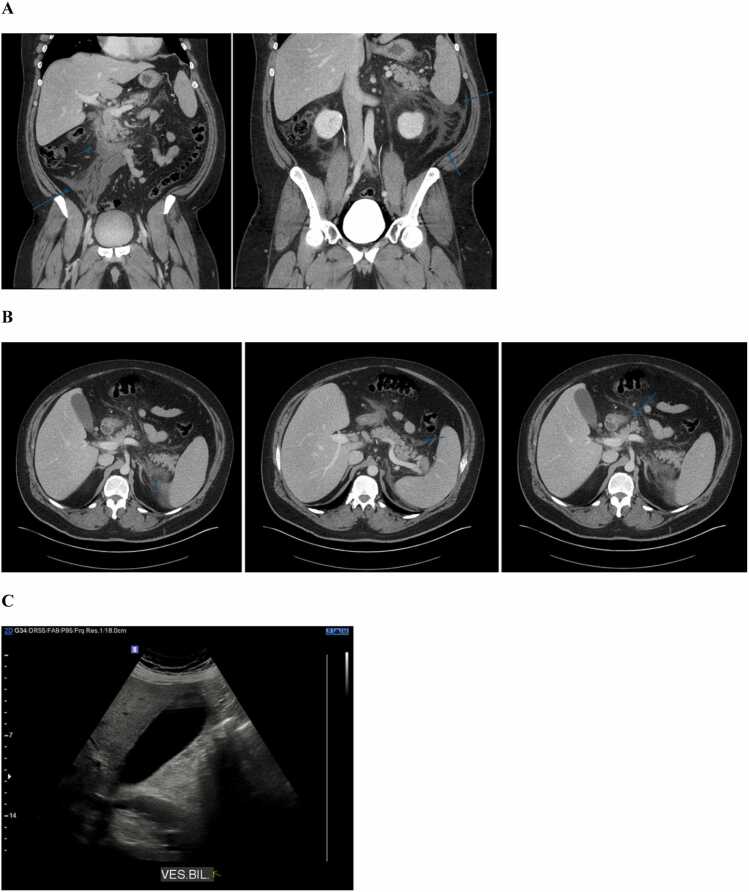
Diagnostic pancreatitis imaging. A Coronal CT view showing fat infiltration in the anterior pararenal space extending into the right iliac fossa and the left flank; B Axial CT view showing peripancreatic fat infiltration, with no venous or arterial thrombosis and no signs of pancreatic necrosis; C Gallbladder ultrasound showing absence of gallstones.
